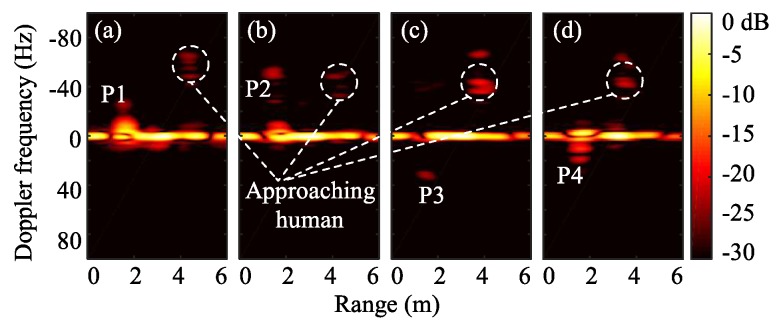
Range-Doppler frames of gesture recognition with the present to two moving targets at the same time. (a) Phase 1; (b) Phase 2; (c) Phase 3; (d) Phase 4 [31].
